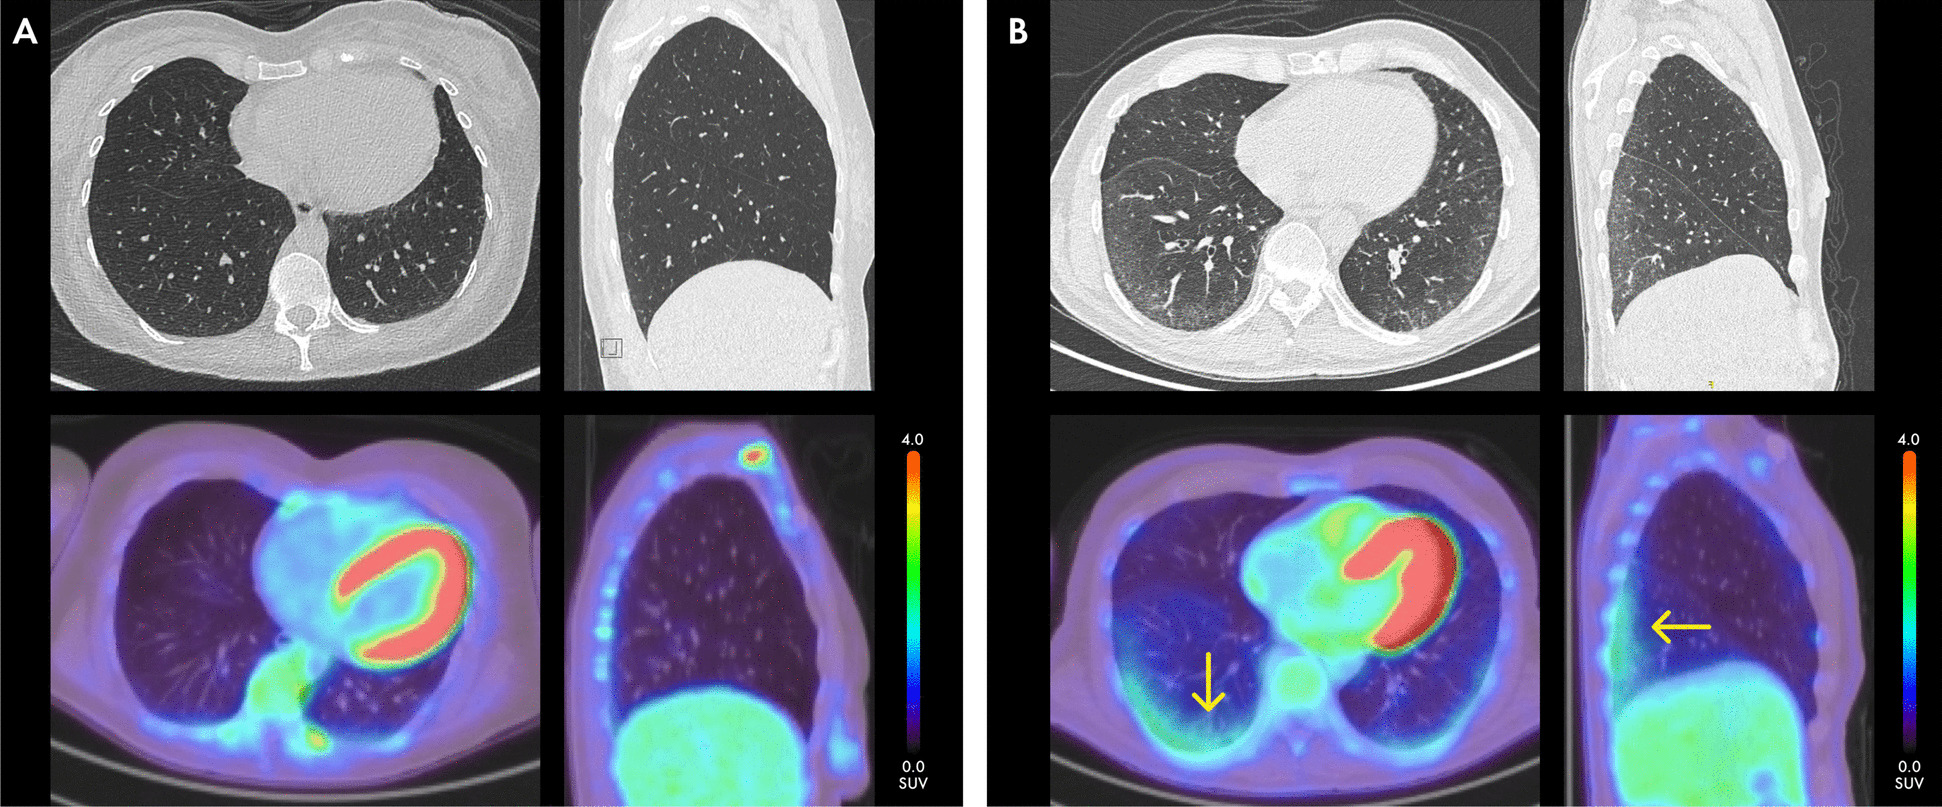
Visual examination of ^18^F-FDG PET-CT and HRCT in SSc-ILD and SSc without ILD. Transverse and sagittal slides of ^18^F-FDG PET-CT and HRCT scans of a SSc patient with (B) and without ILD (A), ^18^F-FDG uptake is indicated by the orange arrows. ^18^F-FDG = ^18^F-Fluorodeoxyglucose; (HR)CT = (High-Resolution) Computed Tomography; ILD = Interstitial Lung Disease; PET = Positron Emission Tomography; SSc = Systemic Sclerosis; SUV = Standardized Uptake Value
